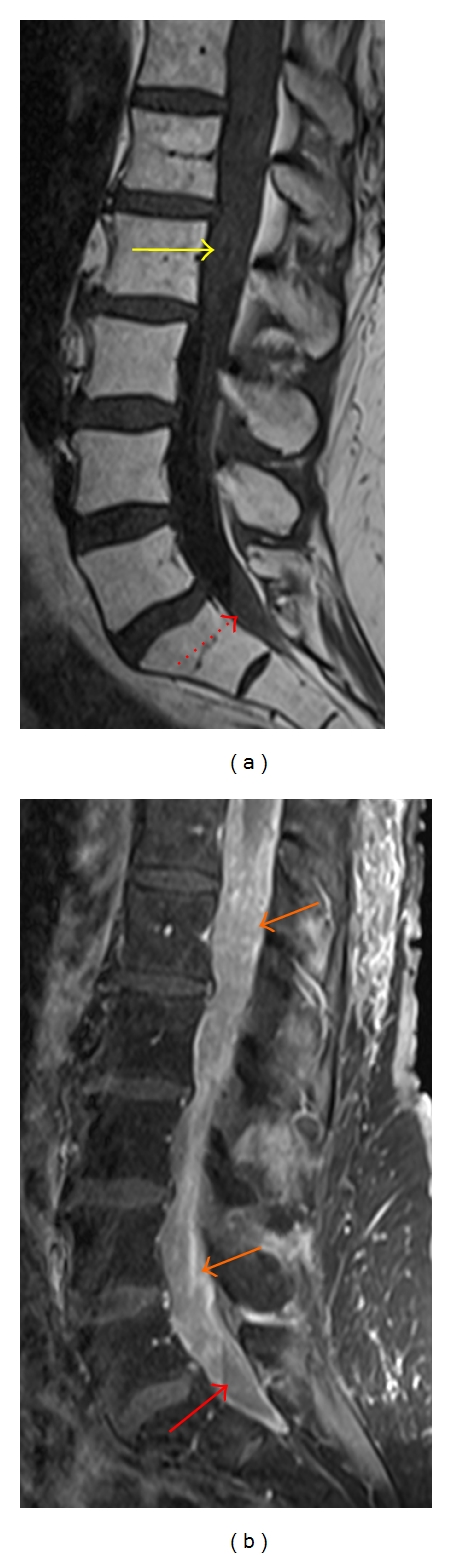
Sagittal T1-weighted MR image (a) shows a hazy appearance of the subarachnoid space (yellow arrow) in the distal thecal sac. Fluid layers dependently in the thecal sac from blood products and/or proteinaceous debris (red arrows). Sagittal enhanced T1-weighted MR image (b) with fat-saturation shows diffuse enhancement of the CSF as well as thick sheet-like enhancement of the cauda equina (orange arrows) related to metastatic melanoma.
